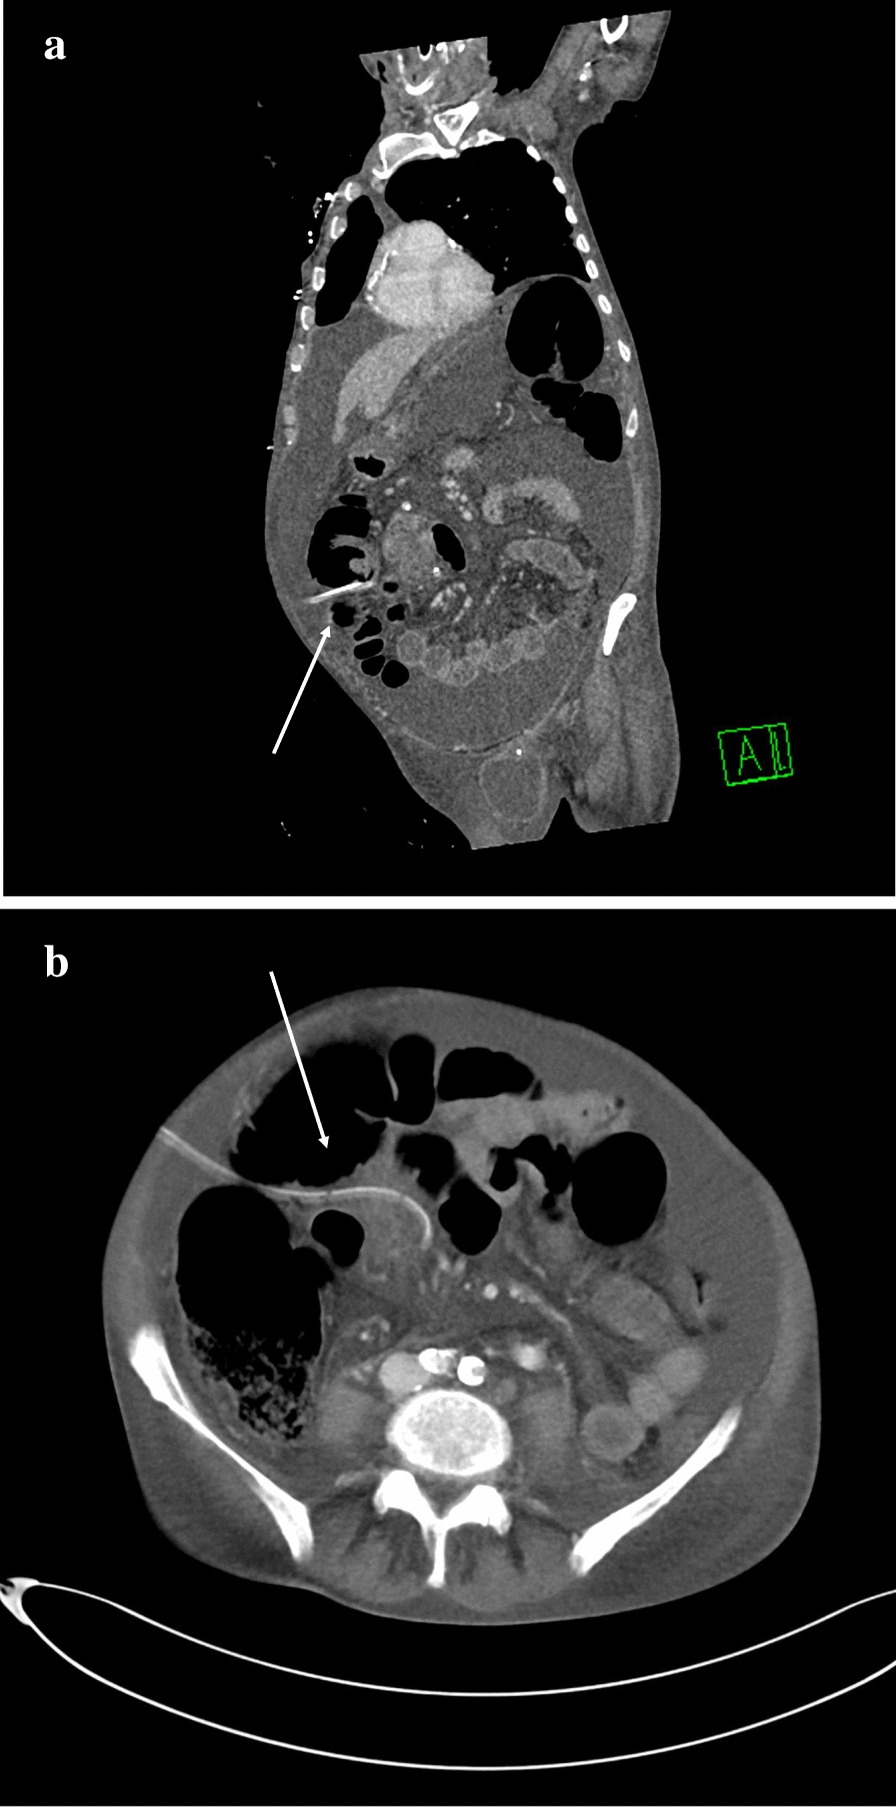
a Coronal computed tomography, showing the entry side of the catheter into the abdominal cavity, next to ascending colon (arrow). b The catheter (arrow) passing through the bowel wall and inserting peritoneal cavity again
